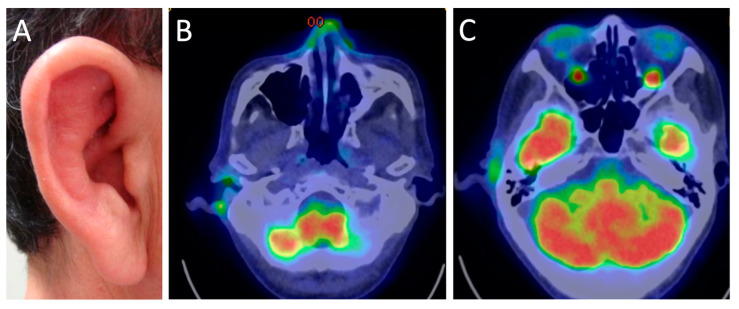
PET/CT images of a patient with right auricular chondritis, nasal chondritis, and bilateral scleritis due to RPC. The right pinna is red and swollen (A), and FDG-PET/CT shows high signal intensities (green) in the right auricle, nasal cartilage (B), and bilateral sclera (C).
